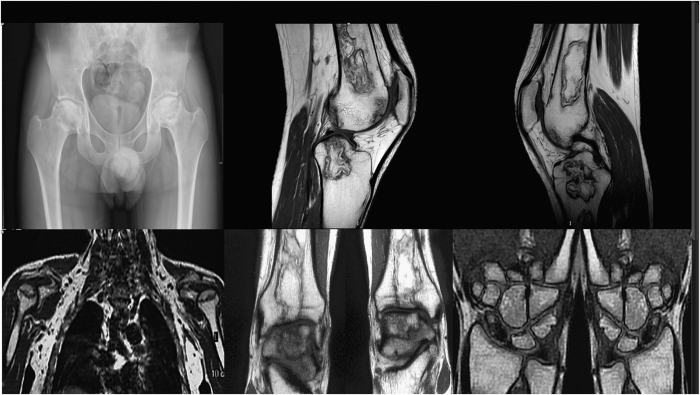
Radiological images of a male patient (age = 22 years). The patient had a history of corticosteroid therapy (equivalent dose of methylprednisolone = 7000 mg) for bone marrow transplantation due to acute lymphoblastic leukemia. He had pain in his hips but no symptoms in his other joints, and he was initially diagnosed with stage IIIc osteonecrosis of the bilateral femoral heads. The MRI examination of his other joints revealed osteonecrosis of the bilateral knees, shoulders, ankles and wrists.
